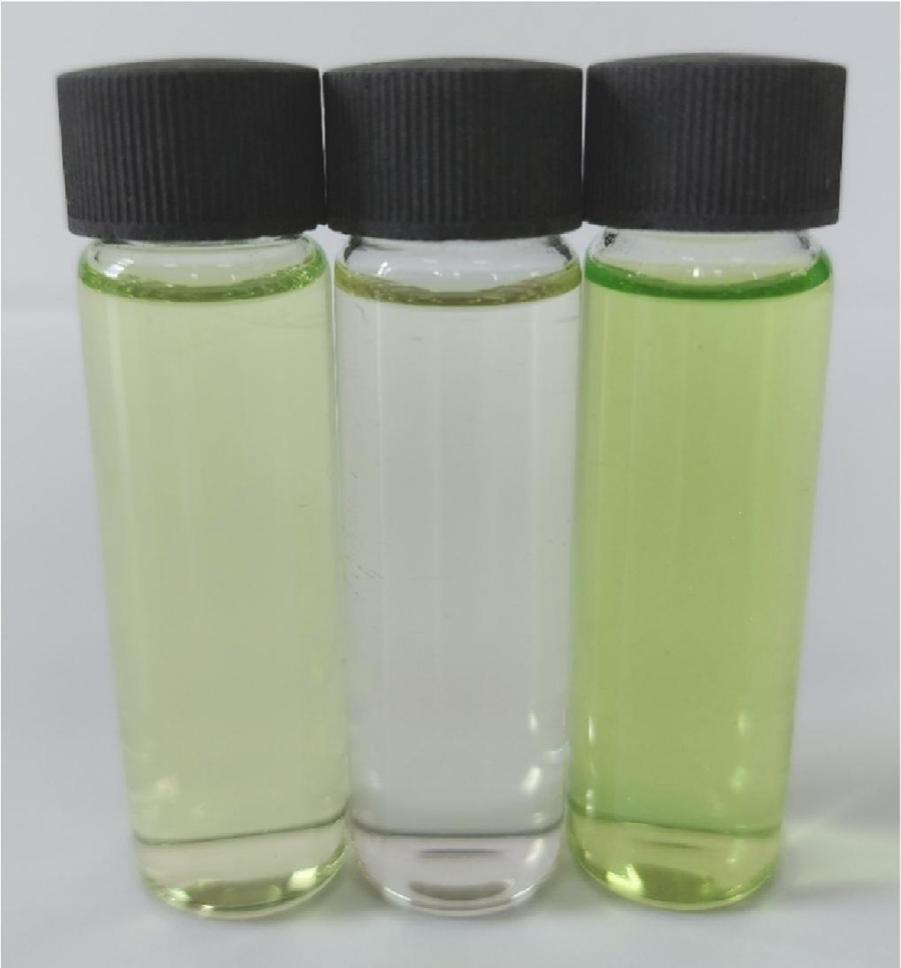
Color change upon mixing TCNQ acceptor (*far left; light green*) with the donor (*middle; colorless*) to generate the CT complex (*far right; intense green*). (For interpretation of the references to color in this figure legend, the reader is referred to the web version of this article.)
